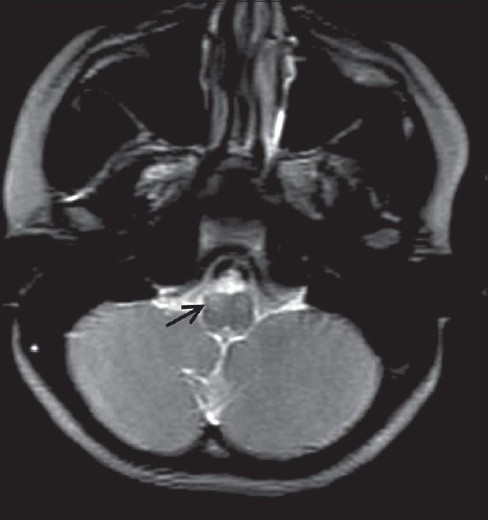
Magnetic resonance imaging T2W axial sequence in a 11-year-old child showing white matter hyperintensity (arrow) in the medulla
